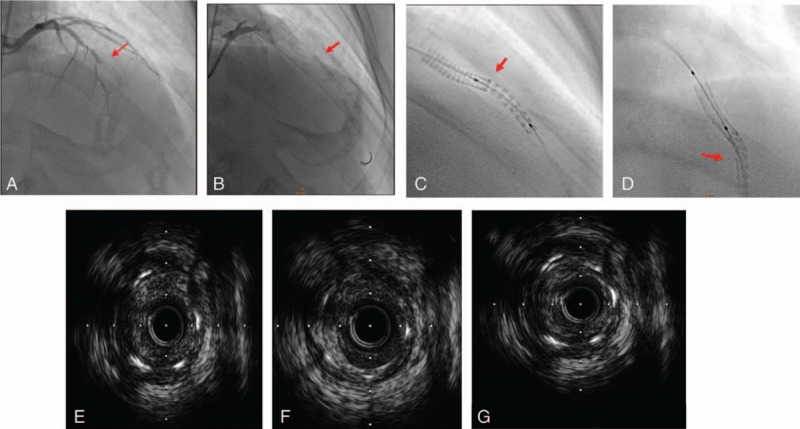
A, Coronary angiography revealed thrombotic occlusion of the stented LAD artery segment (red arrow). B, After thrombus aspiration, coronary angiography showed that the stent struts interrupted close to the overlapping stents (red arrow). C, StentBoost image clearly displayed defected stent struts next to the distal of the 2 overlapped old stents (red arrow). D, Another position showed malalignment of the stent struts (red arrow). E–G, IVUS images showed the stent struts and the decreased number of stent struts from 4 to 5 close to the overlapping of the 2 stents (F). IVUS = intravascular ultrasound, LAD = left anterior descending.
